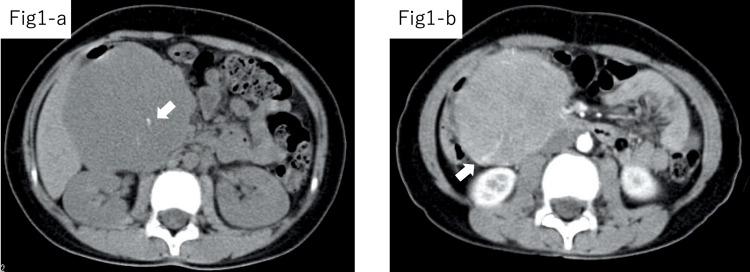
Preoperative CT findings in Case 3. CT revealed microcalcifications in the tumor (1-A, white arrow) and contrast enhancement in the arterial phase (1-B, white arrow) in Case 3. CT: computed tomography
